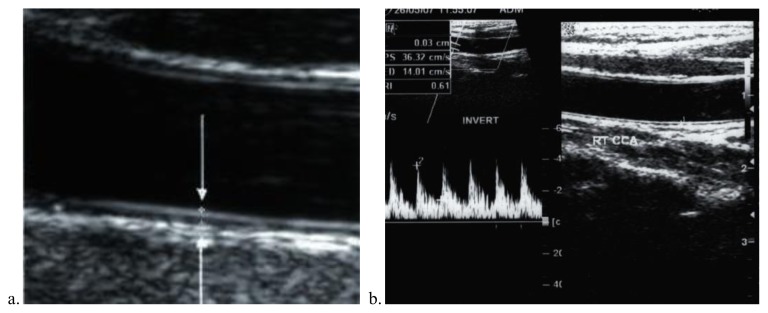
a) Long-axis magnified view of the normal carotid wall anatomy on U/S. The intima and adventia produces echogenic parallel lines (arrows) with an intervening echo void representing the media. b) Long-axis view and Doppler spectrum of the right CCA showing normal intima-media thickness of 0. 3 mm in a 10-year-old healthy child.
